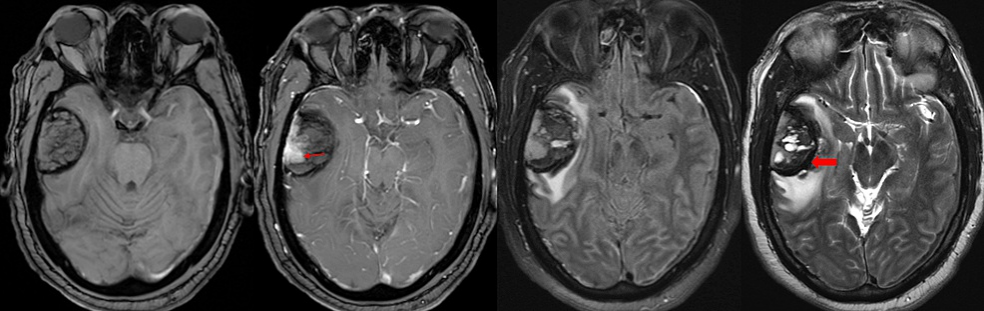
Initial pre- and post-contrast MRI (left to right: T1 pre- and post-contrast axial sequences, FLAIR sequence, and T2-weighted sequence). MRI demonstrating an extra-axial, dural-based mass at the right temporal convexity with nodular, dural-based enhancement (red arrow) with internal hemorrhagic cysts with variable age blood products. Multiple internal hemorrhagic cysts with fluid-fluid levels are present in addition to a well-defined and thickened T2WI hypointense capsule (red block arrow). The absence of a surrounding CSF cleft with prominent, perilesional vasogenic edema, mass effect, and right uncal herniation is also noted.
